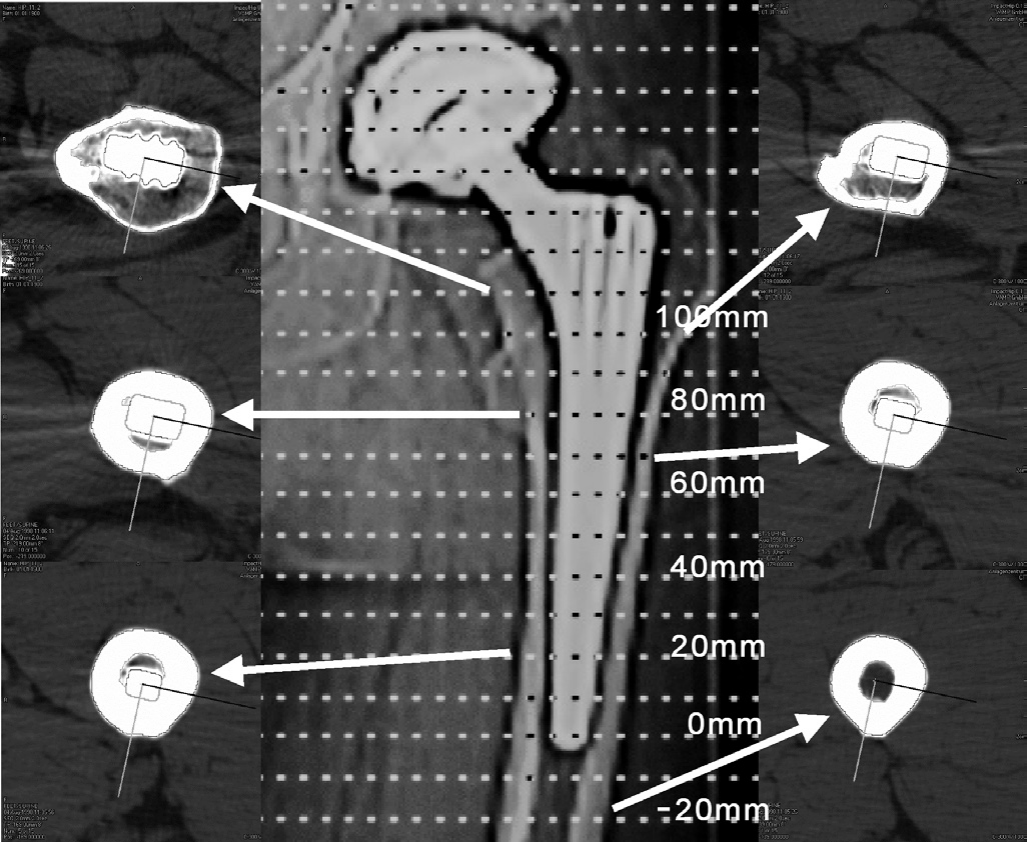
Topogram of an uncemented prosthesis with a selection of CT scans. The first scan of the femur was done 2 cm below the tip of the stem (–20 mm) and the most proximal was done at the 100 mm position.
